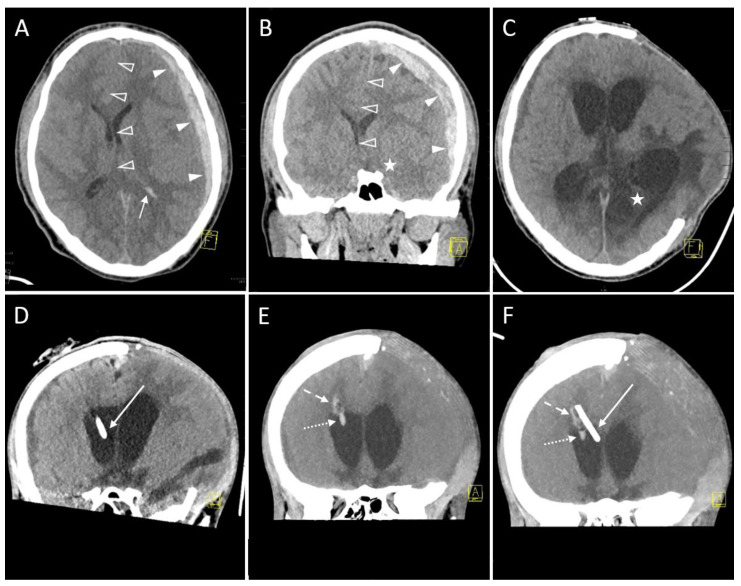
A 16-year-old boy after a motorbike accident. Axial (A) and coronal reformation (B) of the CT scan acquired immediately after the trauma revealed traumatic subdural hematoma (solid arrowheads), midline shift to the right (transparent arrowheads), uncal herniation (asterisk) and traumatic subarachnoid bleeding because of blood collection in the posterior horn of the left ventricle (arrow). A left-sided decompressive trepanation was conducted. Yellow graphic: orientation cube (a: anterior, f: frontal). (C) After one month, a posttraumatic hydrocephalus developed with increasing width of the ventricles. Thus, the indication for an EVD placement was made. Note the infarction of the posterior cerebral artery territory which had occurred due to the herniation and which is now clearly demarcated (asterisk). The left hemispheric brain exceeds the trepanation margins. Onset of general brain atrophy could also be seen. Yellow graphic: orientation cube (f: frontal). (D) Coronal Maximum Intensity Projection (MIP; 4 mm slice thickness) of the control scan after EVD placement shows the tip in the frontal horn of the right ventricle (Kakarla I). Yellow graphic: orientation cube (a: anterior). (E) However, after 3 days the EVD has been removed due to clotting (dotted arrow) and intraparenchymal hemorrhage (dashed arrow; volume ≥ 1 to 15 mL, Grade 2 according to Wiesmann and Mayer). Yellow graphic: orientation cube (a: anterior). (F) Coronal Maximum Intensity Projection (MIP; 20 mm slice thickness) of the control scan after placement of the new EVD. Its tip (solid arrow) is located near the septum pellucidum (Kakarla I). Clotting (dotted arrow) and intraparenchymal hemorrhage (dashed arrow) caused by the former EVD are still visible. Yellow graphic: orientation cube (a: anterior).
